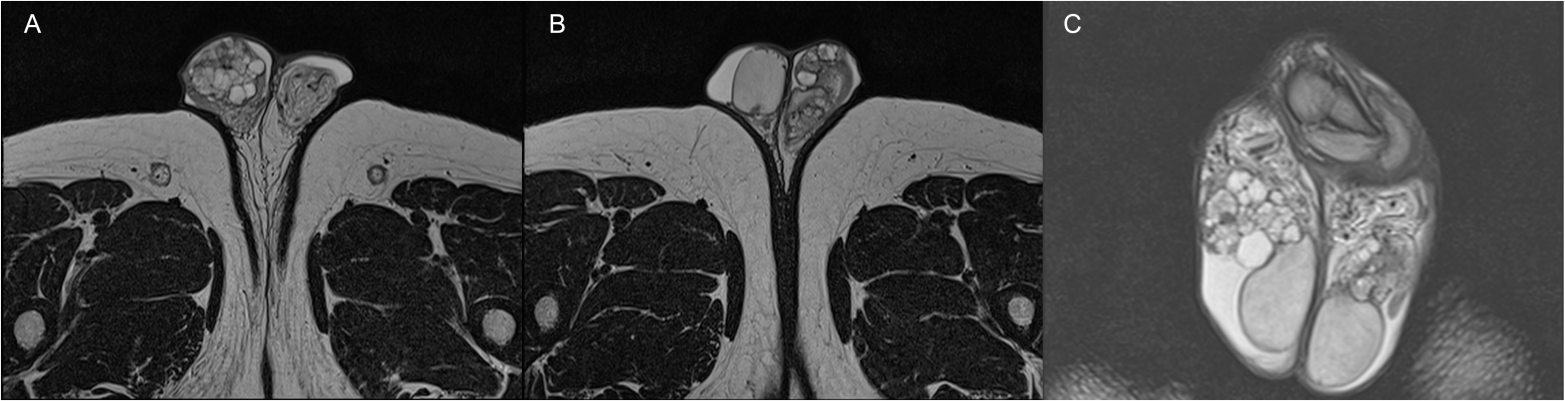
Axial (A, B) and coronal (C) TSE T2-weighted MRI images showing the presence of bilateral papillary cystadenoma of the epididymis.
